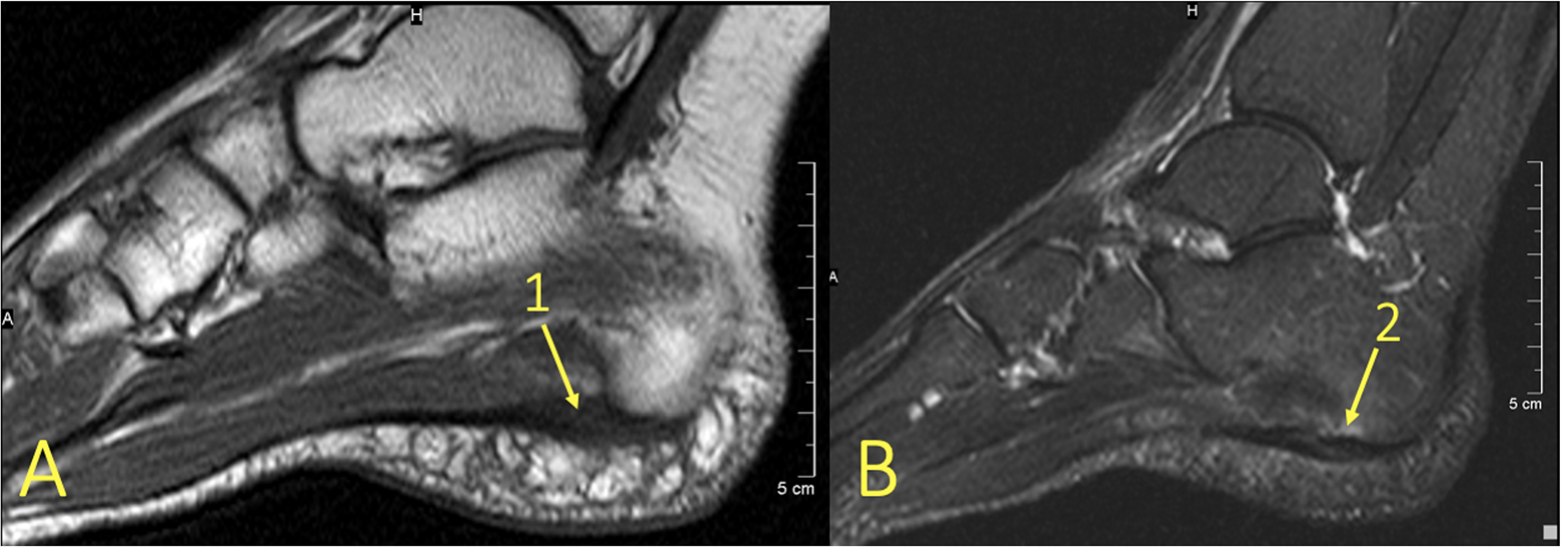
A 43-year-old female with chronic pain in the plantar part of the heel and suspicion of a heel spur and plantar aponeurosis rupture. MRI revealed (1) enthesopathy of the plantar fascia with (2) bone marrow edema in the calcaneus
